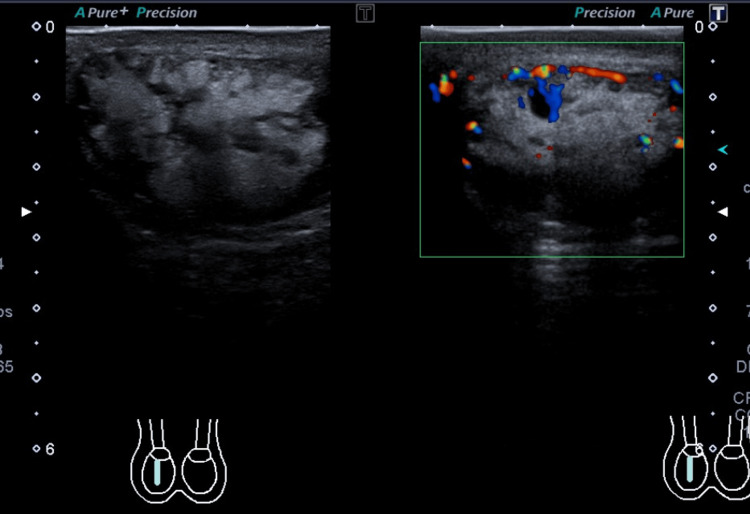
Scrotum ultrasound of the 30-year-old male.
